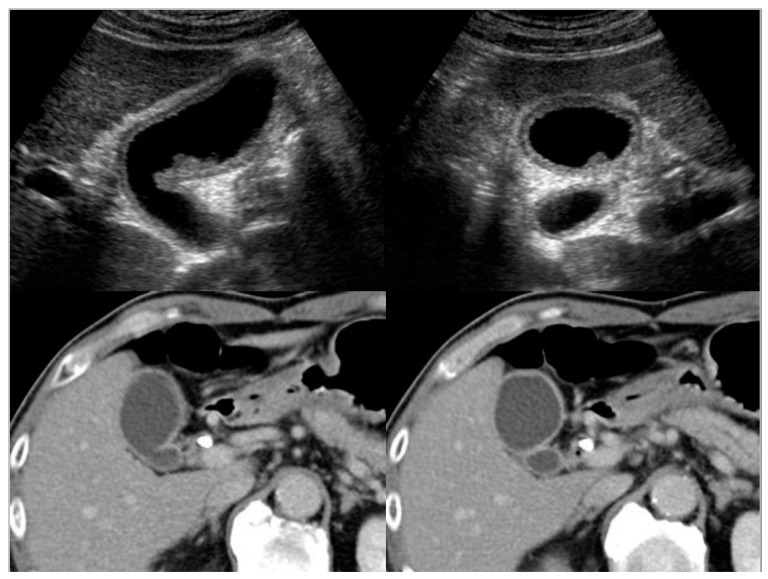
US (3-layer type) and CT (1-layer type) findings of a case with a diffuse type of IgG4-sclerosing cholecystitis.
